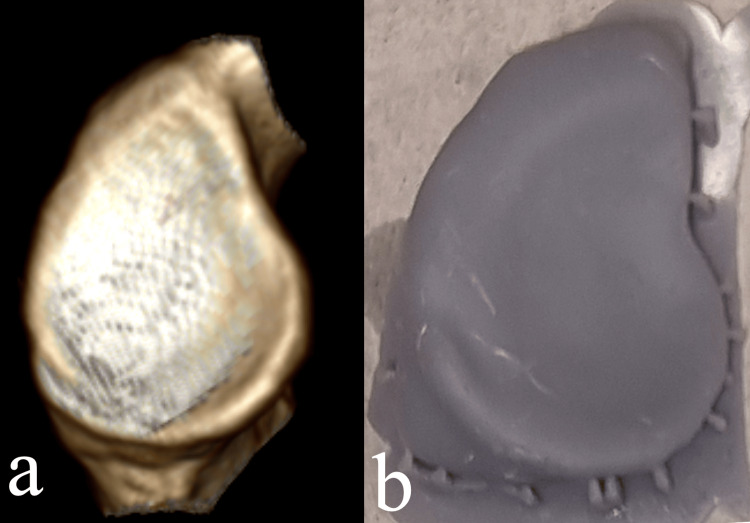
Visual comparison of the 3D CT reconstructed glenoid models and the 3D printed models. On visual inspection, there is no difference in the morphology of the 3D CT reconstructed glenoid models (a) and the 3D printed models (b).
